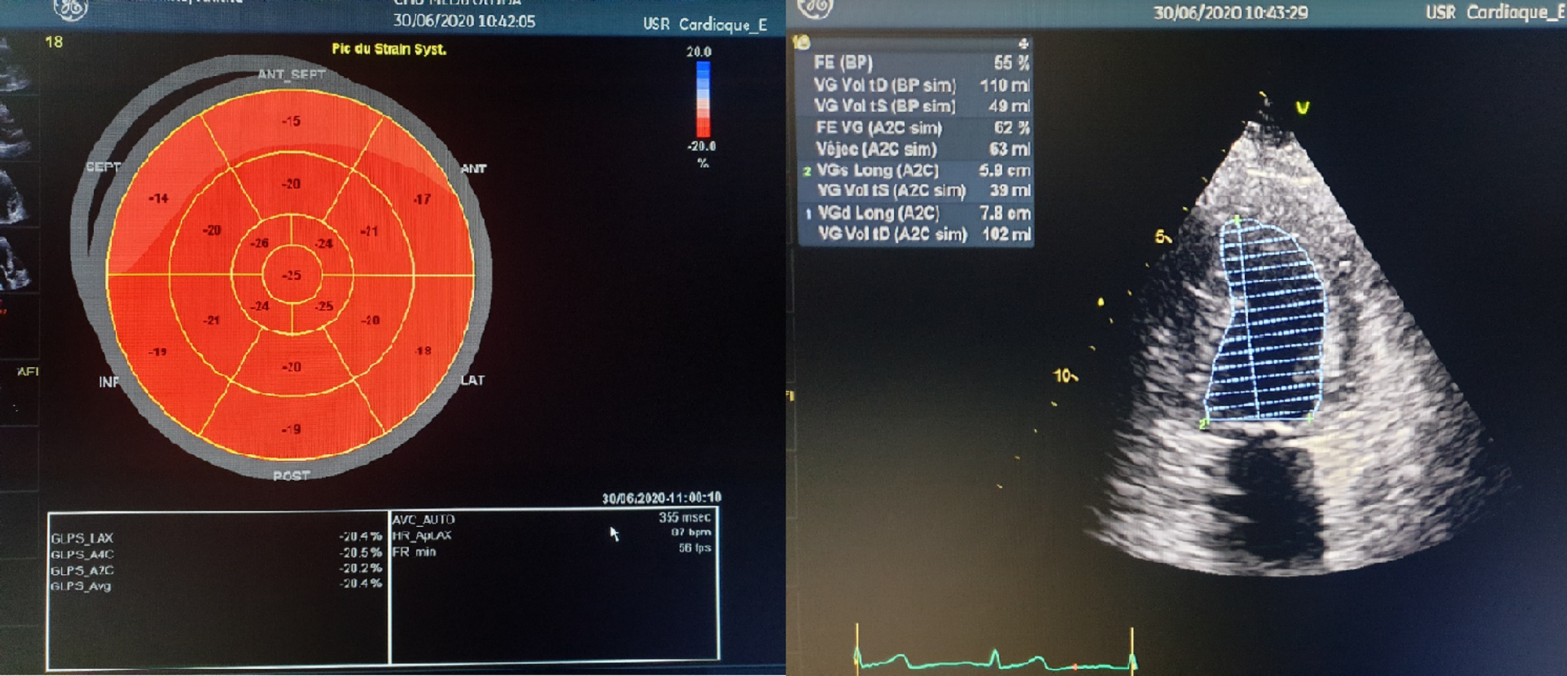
Echocardiography showing recovery of normal LVEF LVEF - left ventricular ejection fraction
